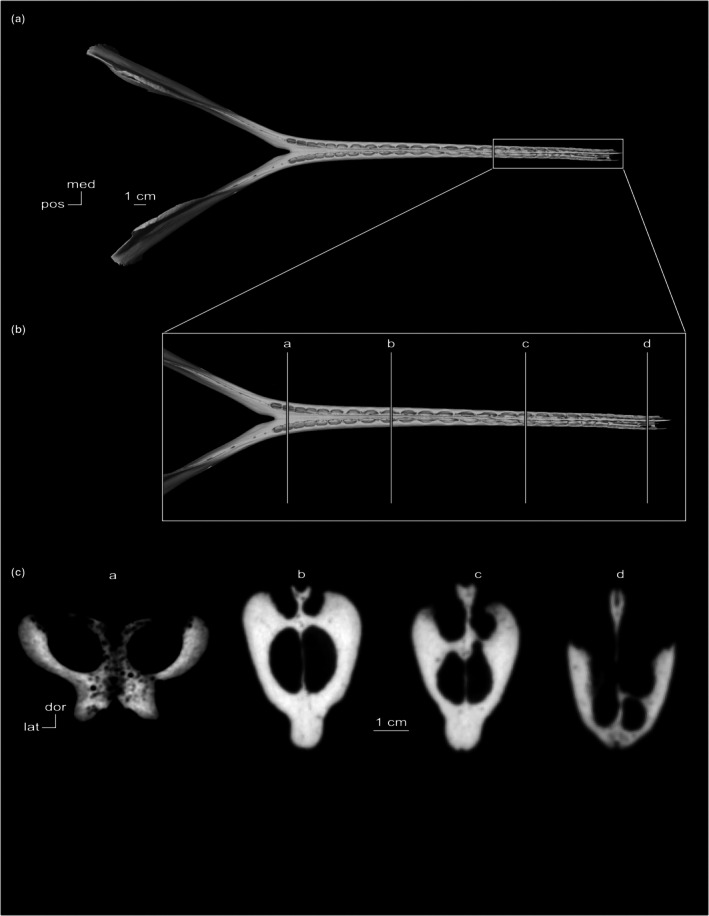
Mandibles of *Platanista gangetica* (USNM VZ 23456). (a) Dorsal view. (b) Close‐up of the symphysis in dorsal view showing the level of CT cross‐sectional slices (a–d). (c) CT coronal cross‐sectional slices (a–d).
